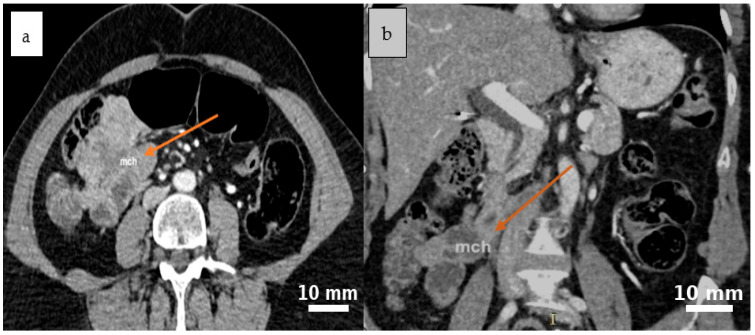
Post-contrast CT scan reveals a cystic lesion situated between the intestinal loops in the ileocecal region, characterized by mild peripheral attachment and slightly heterogeneous internal content, as seen in the axial (a) and coronal (b) planes. Yellow arrows indicate the cystic lesion (*mch = mesenteric cystic hygroma—lymphatic malformation*).
